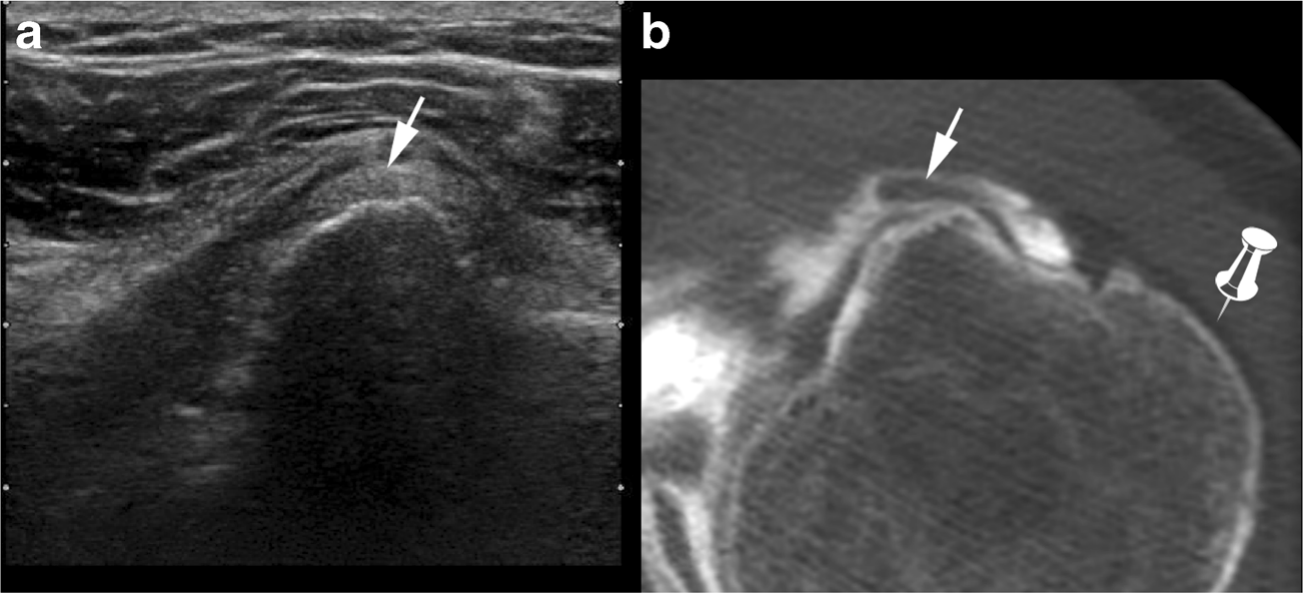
Ultrasound (a) and computed tomography (CT) arthography (b) correlation: complete partial thickness tear of the upper SSC tendon responsible for subluxation of the LHBT (white arrow), located in front of the medial edge of the intertubercular sulcus. On CT-arthrography (b), contrast in the intertubercular groove is in direct continuity with intra-articular contrast, without any opacification of the SASD bursa (pin) because the bursal side of the transverse ligament is intact
